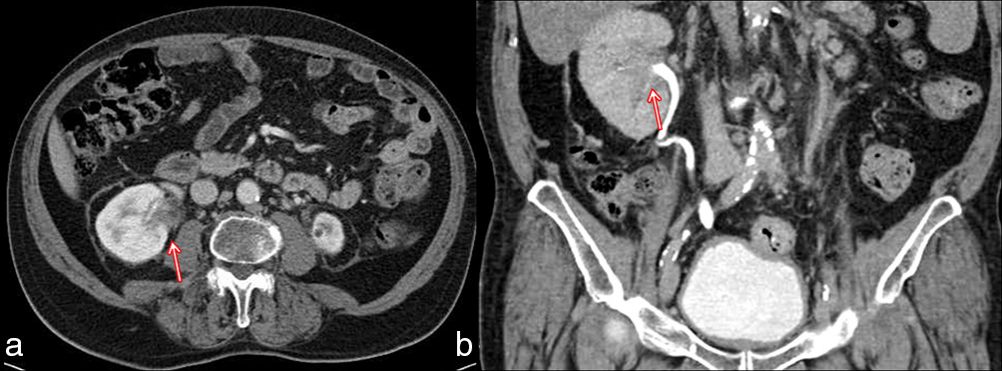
(a) Axial portal-phase CT scan performed 1 year later showing new solid tissue surrounding the right renal pelvis and calices (red arrow). (b) Coronal delayed-phase CT image confirming the presence of solid tissue infiltrating the renal pelvis (red arrow).
